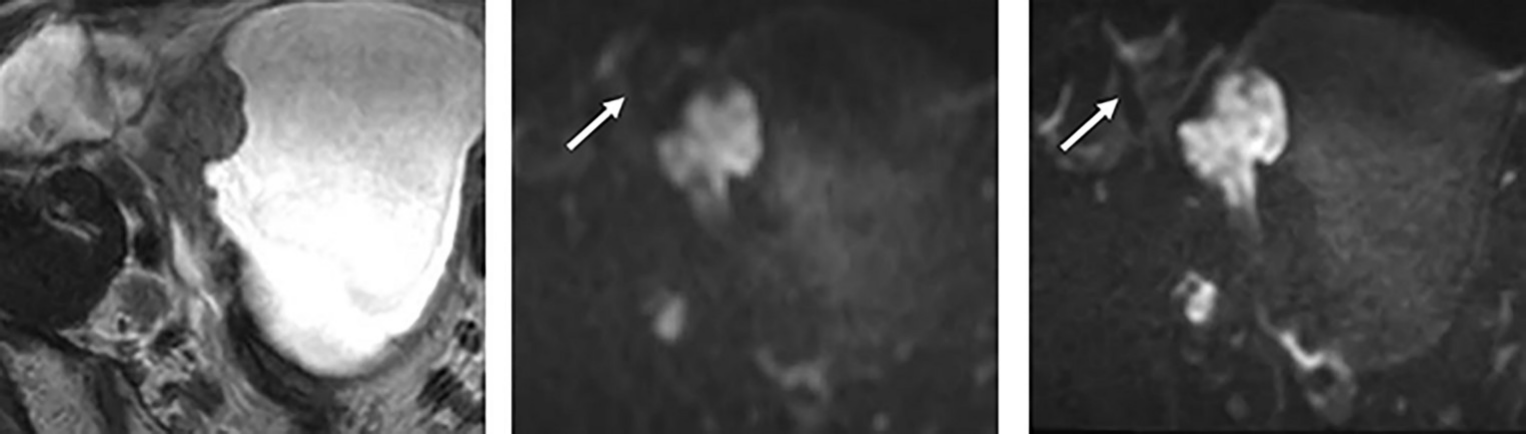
53-year-old man with the urothelial carcinoma of pT2 or more on the right side wall of the bladder. (A) T2WI (B) fFOV DWI (C) Zoomed DWI. The bladder wall demonstrated more clearly on Zoomed DWI than on fFOV DWI. An artifact appears from the intestine and the rectum in both images of fFOV and Zoomed DWI. VI-RADS score was 5 for both readers.
